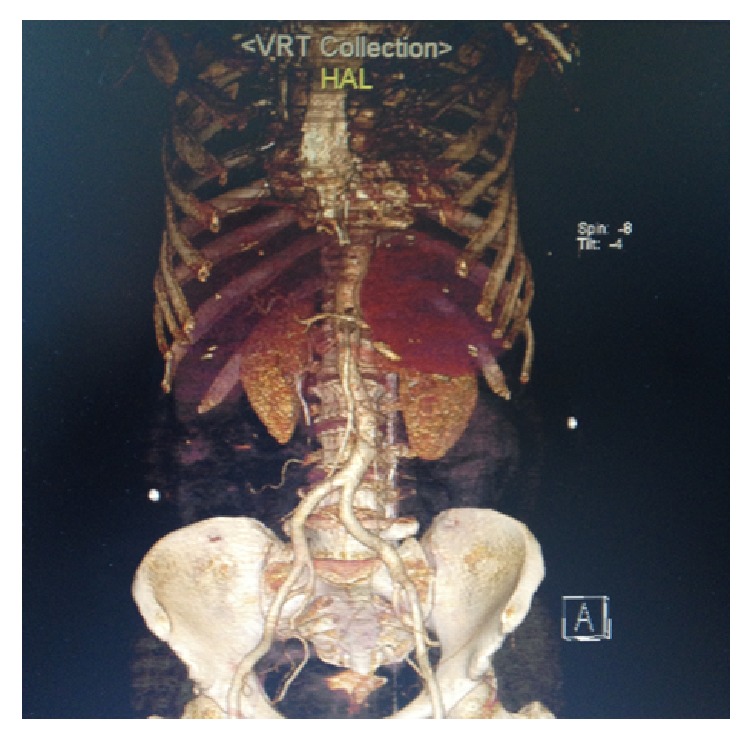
Computed tomographic angiography revealed aneurysmatic expansion.
